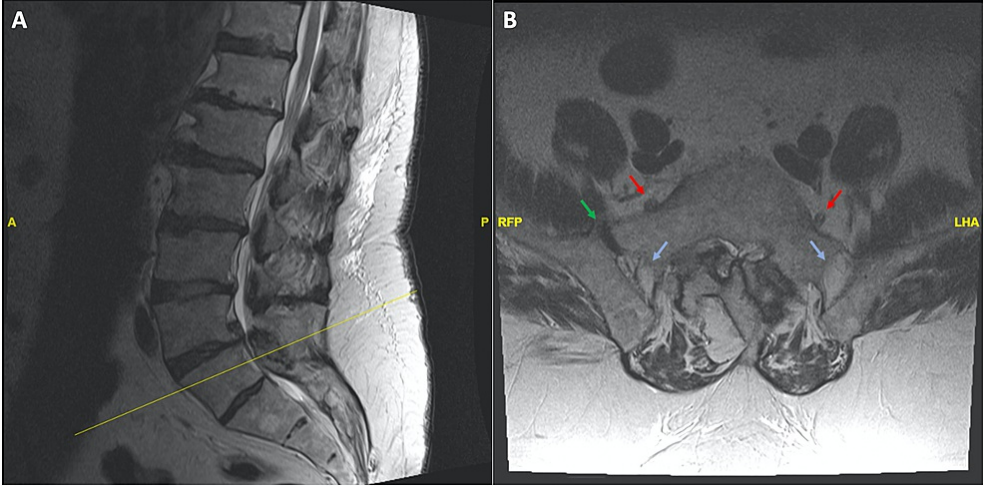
MRI of lumbar spine: (1A) T2-weighted sagittal scan and (1B) T2-weighted axial scan showing L5 nerve roots (red), ligaments (blue), and anterior joint space (green). Incidental hemangioma seen in S2 vertebral body.
